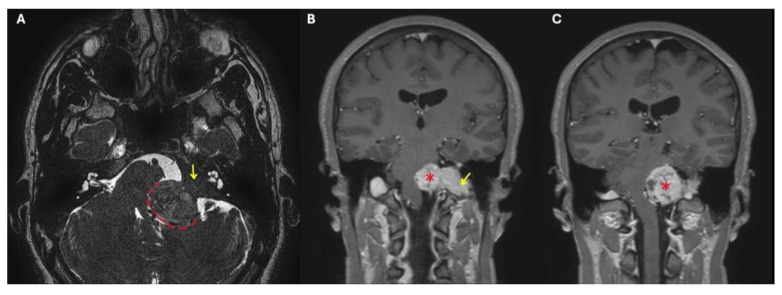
Brain and cerebellopontine-angle MRI of a TJ-PG Di2 class. (A): T2-weighted image; (B) and (C): T1-weighted images with contrast enhancement. *: intradural portion of the TJ-PG Di2; dashed line: brainstem compression by the TJ-PG Di2 in the cisterna magna; yellow arrow: extradural portion of the TJ-PG Di2.
